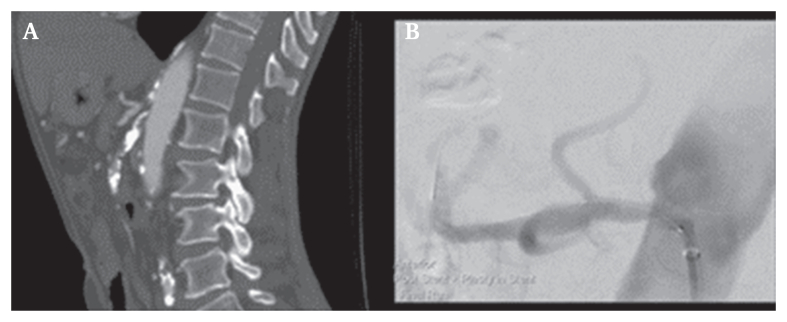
Computed tomography angiography images. A. Stenosis of the coeliac artery and occlusion of the superior mesenteric artery. B. Completion run post-coeliac artery stenting.
